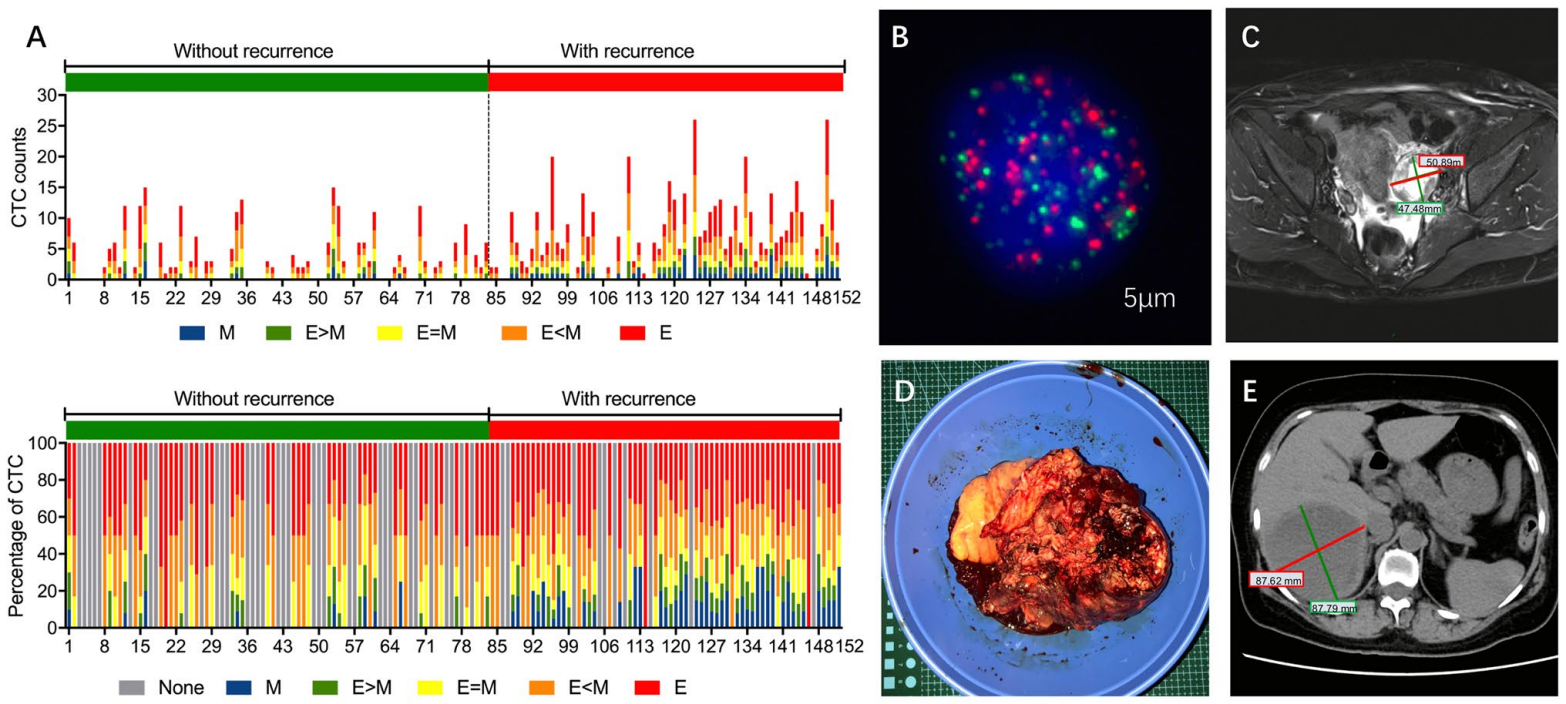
(A) Total CTC counts (top) and percentage of each CTC category (bottom) before treatment in ovarian cancer patients with or without recurrence. (B) The CTC image and (C) Magnetic Resonance Imaging (MRI) image of a representative patient who had the CTC-positive blood sample before treatment. (D) After tumor resection, the patient suffered cancer recurrence with (E) detectable liver metastasis observed by Magnetic Resonance Imaging (MRI) at 6-month follow-up.
